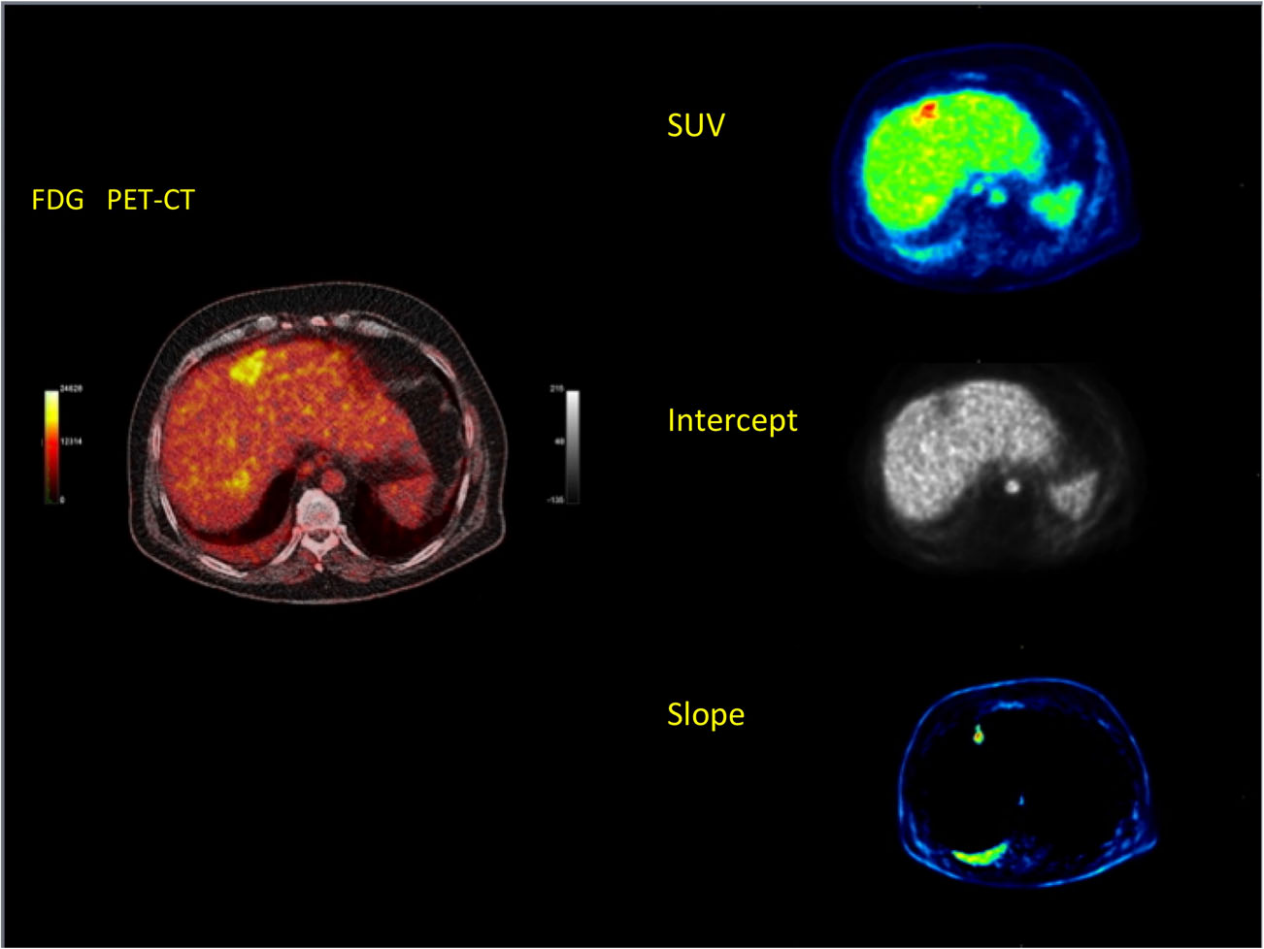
Patient with a liver metastasis of rectal cancer following FOLFOX chemotherapy. Fused transversal ^18^F-FDG PET/CT image (left) demonstrating enhanced uptake at the site of the metastasis 60 min p.i. Transversal SUV image 50–60 min p.i. demonstrates an enhanced uptake (right upper row), while parametric image of the intercept (middle row) shows a decrease in the perfusion-related ^18^F-FDG uptake, and parametric image of the slope (lower row) demonstrates an enhanced phosphorylation-related ^18^F-FDG uptake
